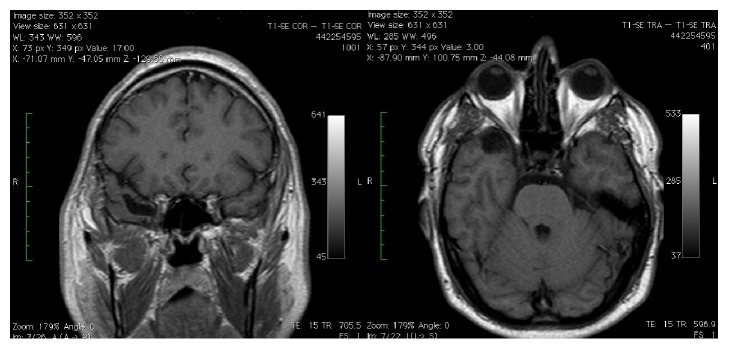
An MRI scan performed 5 months after surgery shows complete removal of the tumour.
